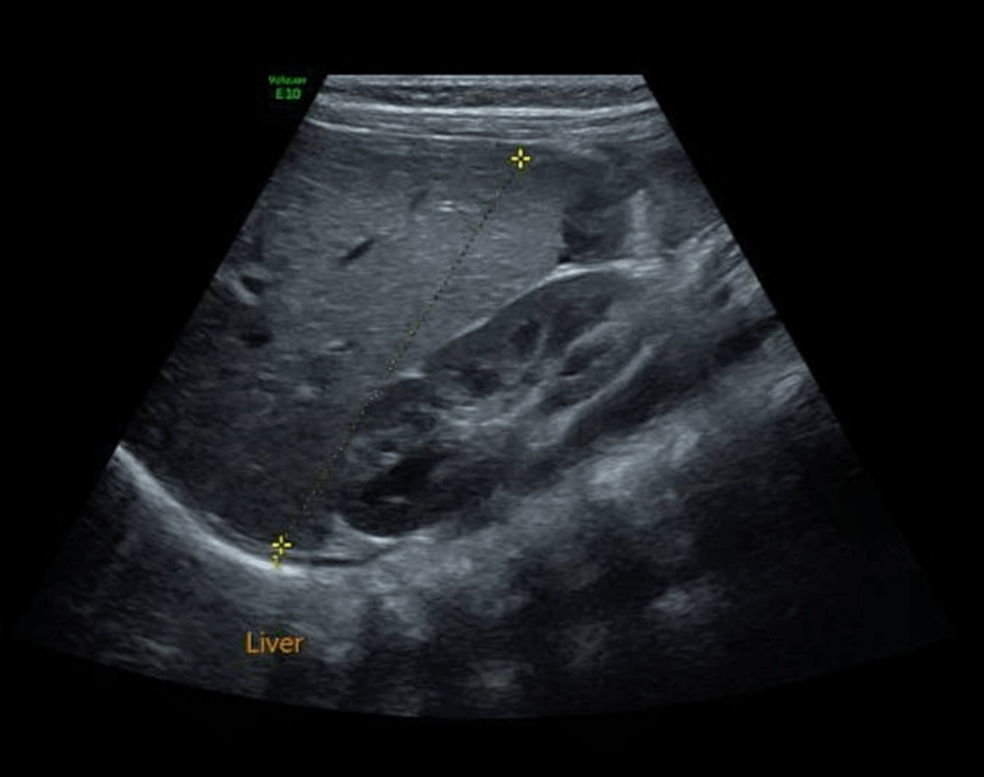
Normal ultrasound abdomen
